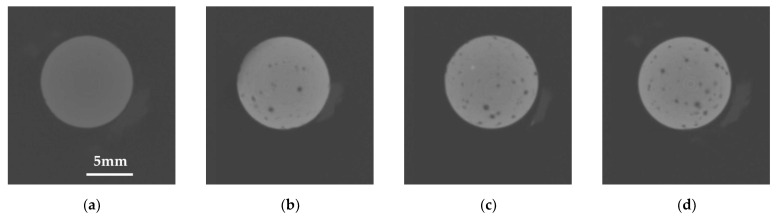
Micro CT images of the resin discs; (a) control (SAR); (b) SAR + 1% MBN; (c) SAR + 3% MBN; and (d) SAR + 5% MBN.
